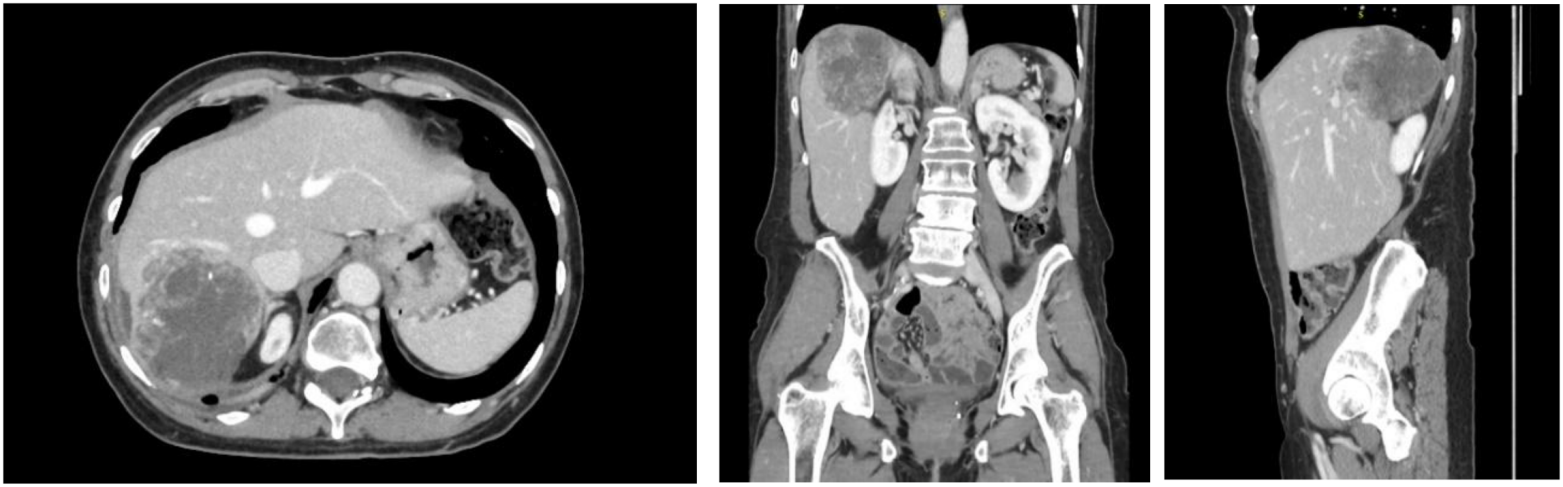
CT AP with contrast 5/29/2024: Decreased hepatic mass now 7.7 x 6.3 cm. Stable upper retroperitoneal adenopathy.
